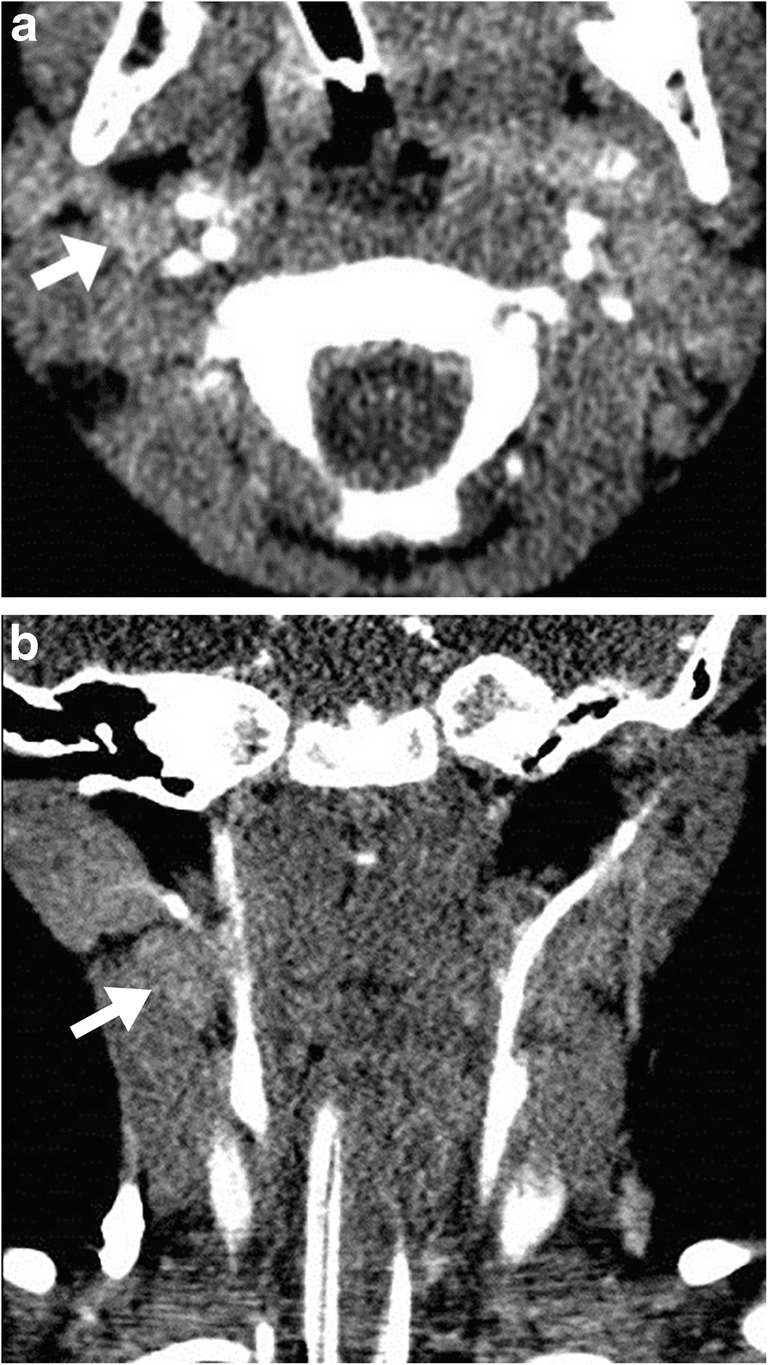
Cervical contrast-enhanced CT images (100 kV, 70 mAs) in a 2-year-old boy. a Axial reconstruction shows a Level II lymph node (*arrow*) with a short-axis diameter of 8 mm and a long-axis diameter of 10 mm. b Corresponding coronal image shows the same Level II lymph node (*arrow*) with a long-axis diameter of 16 mm. The depicted images provide an example of the vertical alignment of lymph nodes that is often found among cervical lymph node stations
